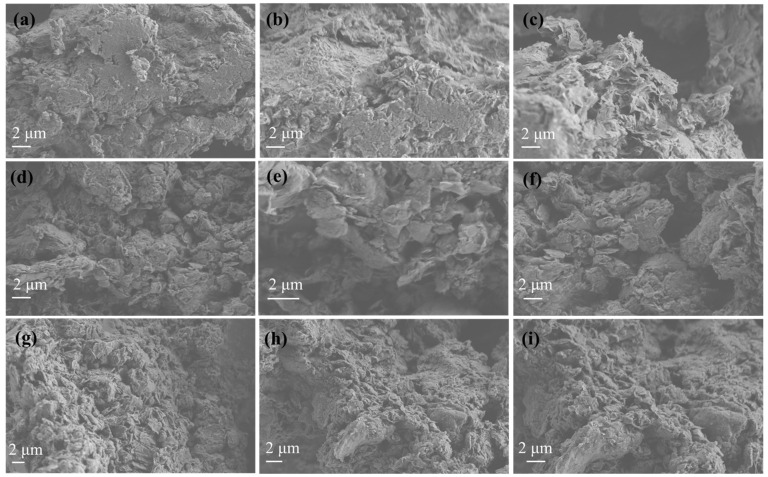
HR-SEM images of (a–c) pure Mt, (d–f) EG@Mt nanohybrid, and (g–i) CT@Mt nanohybrid.
